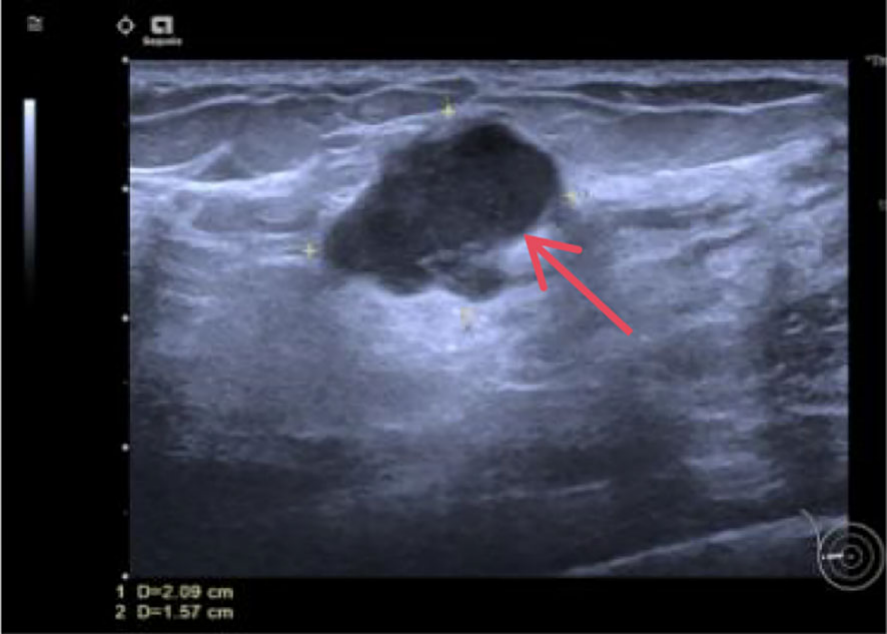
Color doppler ultrasound: A 2.1 x 1.6 x 1.6 cm irregularly shaped solid hypoechoic mass is noted at the 9 o’clock position in the right breast, approximately 3.7 cm from the nipple and 0.5 cm from the skin surface. The aspect ratio exceeds 1. The margins are ill-defined with a spiked appearance. Internal echoes are heterogeneous, showing multiple small punctate hyperechoic foci. Posterior acoustic attenuation is present. with a surrounding hyperechoic halo. CDFI: Rich blood flow is visible within the lesion. BI-RADS: Category 5 (the red arrow in the image points to the mass).
